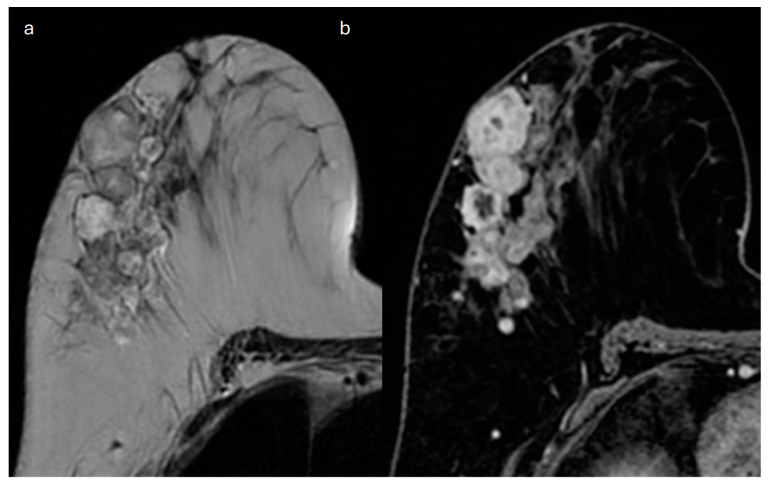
Breast MRI of a 45-year-old patient with a mucinous carcinoma of the right breast. (a) Axial T2-weighted MRI image shows multiple mass-like lesions tightly bound one to each other, one in continuity with the skin, characterized by internal areas of high signal intensity. (b) Axial T1-weighted fat-suppressed contrast-enhanced dynamic image demonstrates a heterogeneous internal enhancement.
